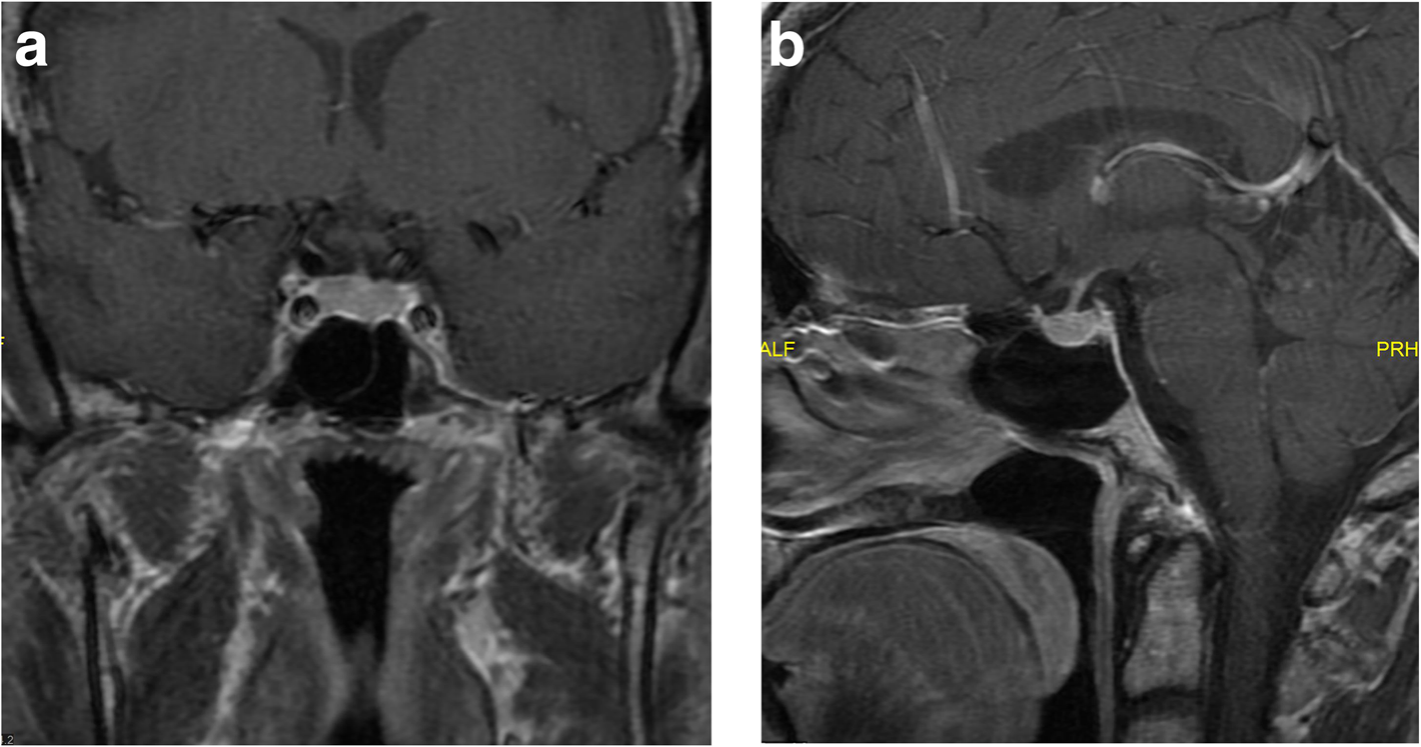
Pituitary MRI Coronal View. a Coronal view. b Sagittal view
